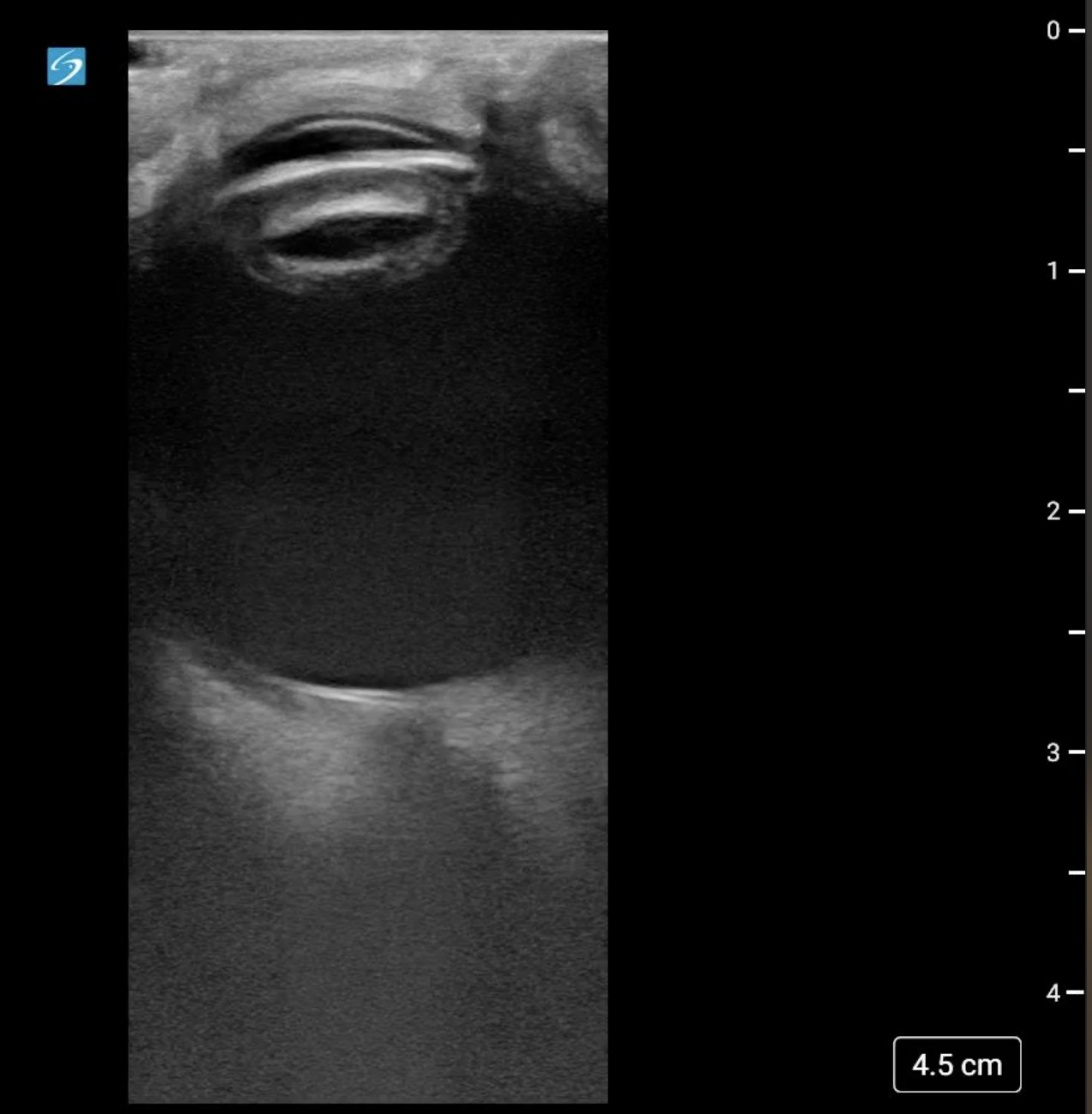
Ocular point of care ultrasound (POCUS) image of the patient's affected eye. Lens is irregular and thickened with increased echogenicity.
